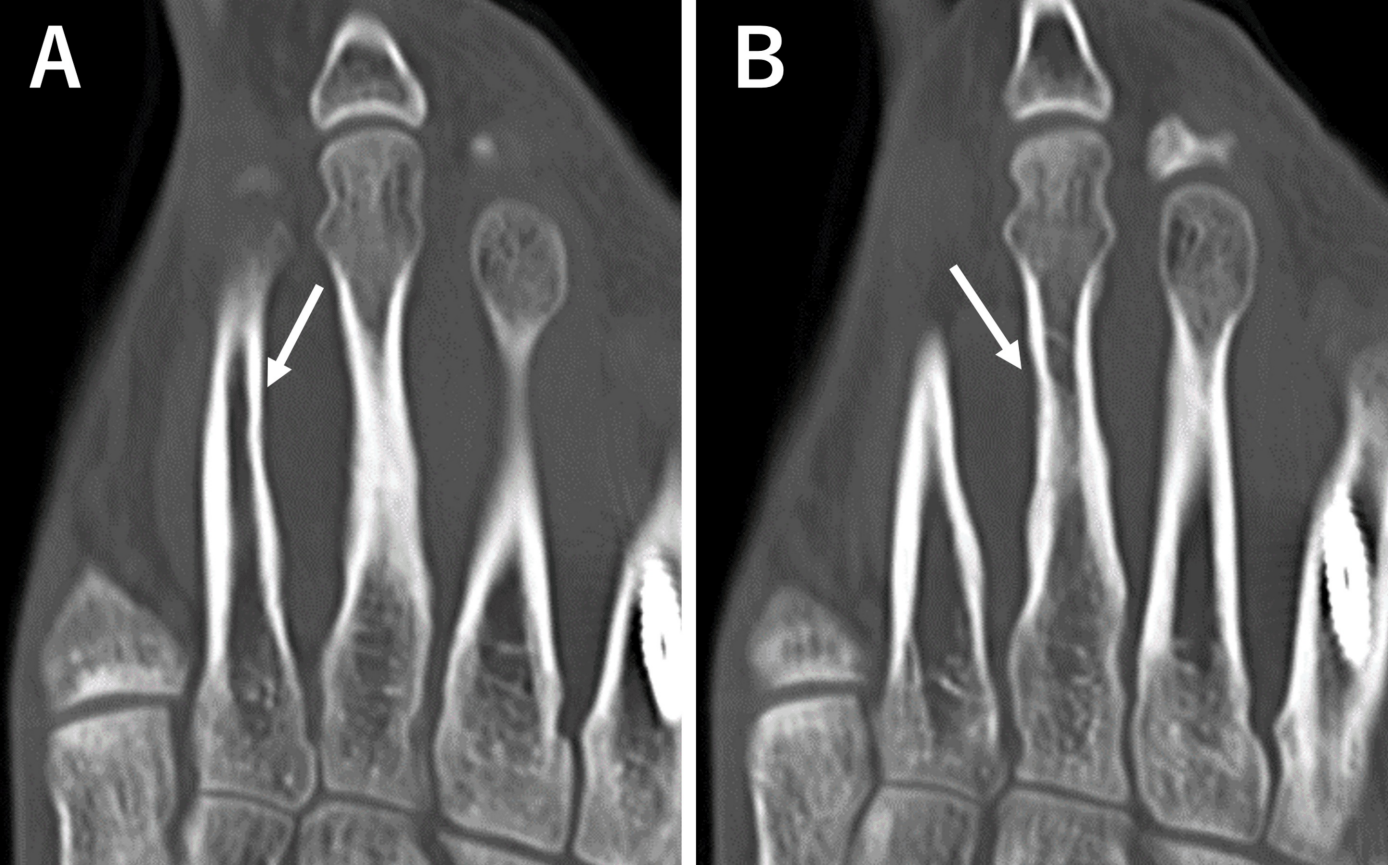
CT showing bone cortex thinning in the second (A) and third (B) metatarsals.
